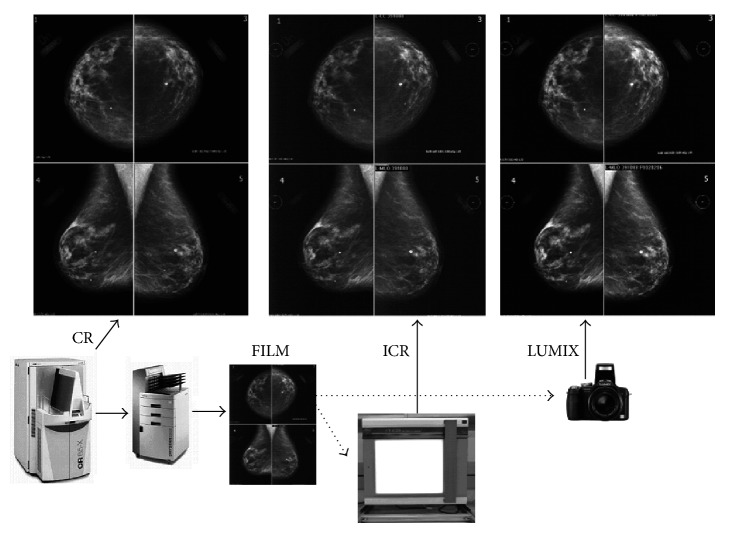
Digital image and film generation. CR: computed radiography; FILM: printed film; LUMIX: Lumix DMC-FZ28 digital camera; ICR: iCR 612SL specialized digitizer.
